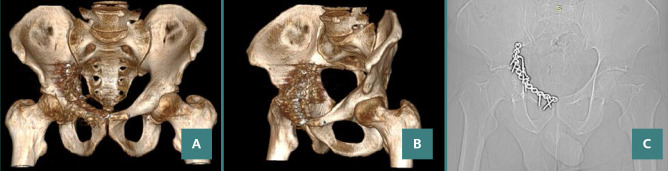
One-year postoperative imaging results with CT (A,B) and X-Ray (C)
